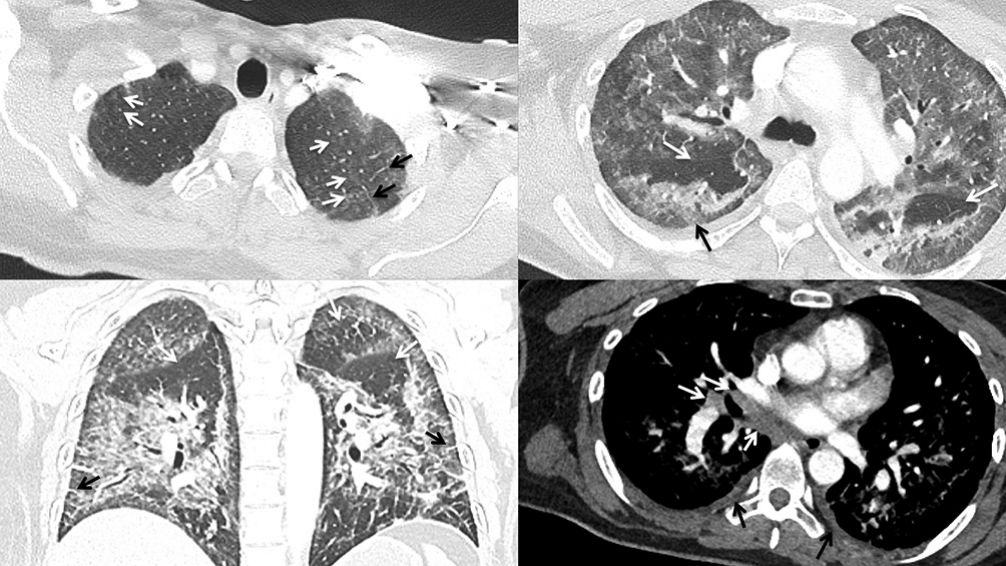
A (top left). Axial HRCT image showing mild centrilobular groundglass opacities in the both apices (white arrows) and scant interlobular septal thickening at the left apex (black arrows) B (top right). Axial HRCT image showing bilateral geographic airspace opacities with lobular (white arrows) and subpleural (black arrow) sparing C (bottom left). Coronal CT image showing bilateral lower lobe predominant airspace opacities with lobular sparing (white arrows) and mild septal thickening (black arrows) D (bottom right). Axial CT image showing mild subcarinal and right hilar lymphadenopathy (white arrows). HRCT, high-resolution CT.
